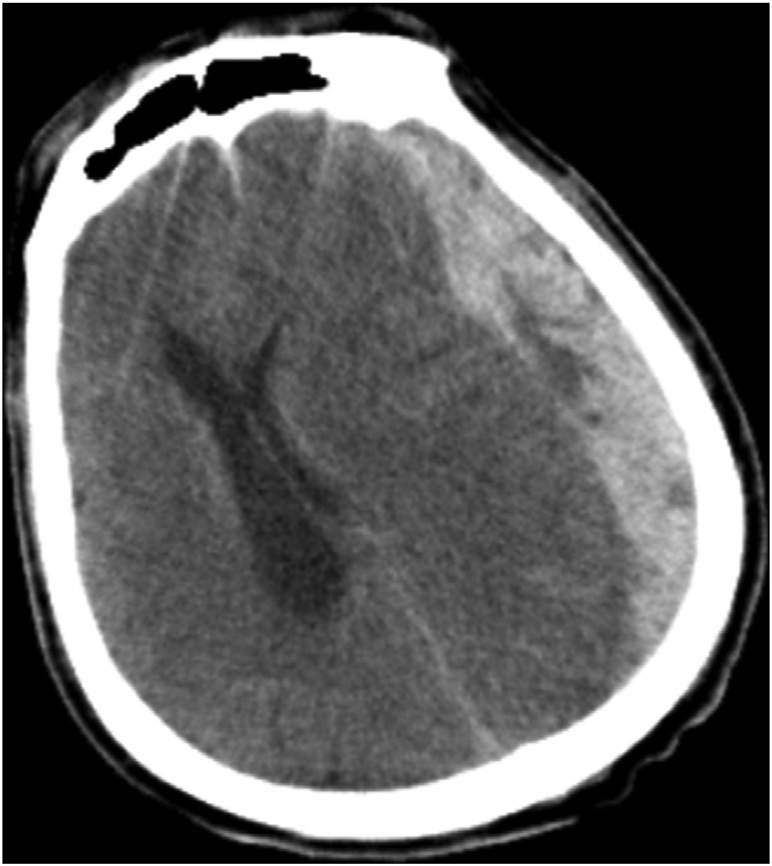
Brain CT scan, showing left fronto-temporo-parietal subacute haematoma with midline shift.
